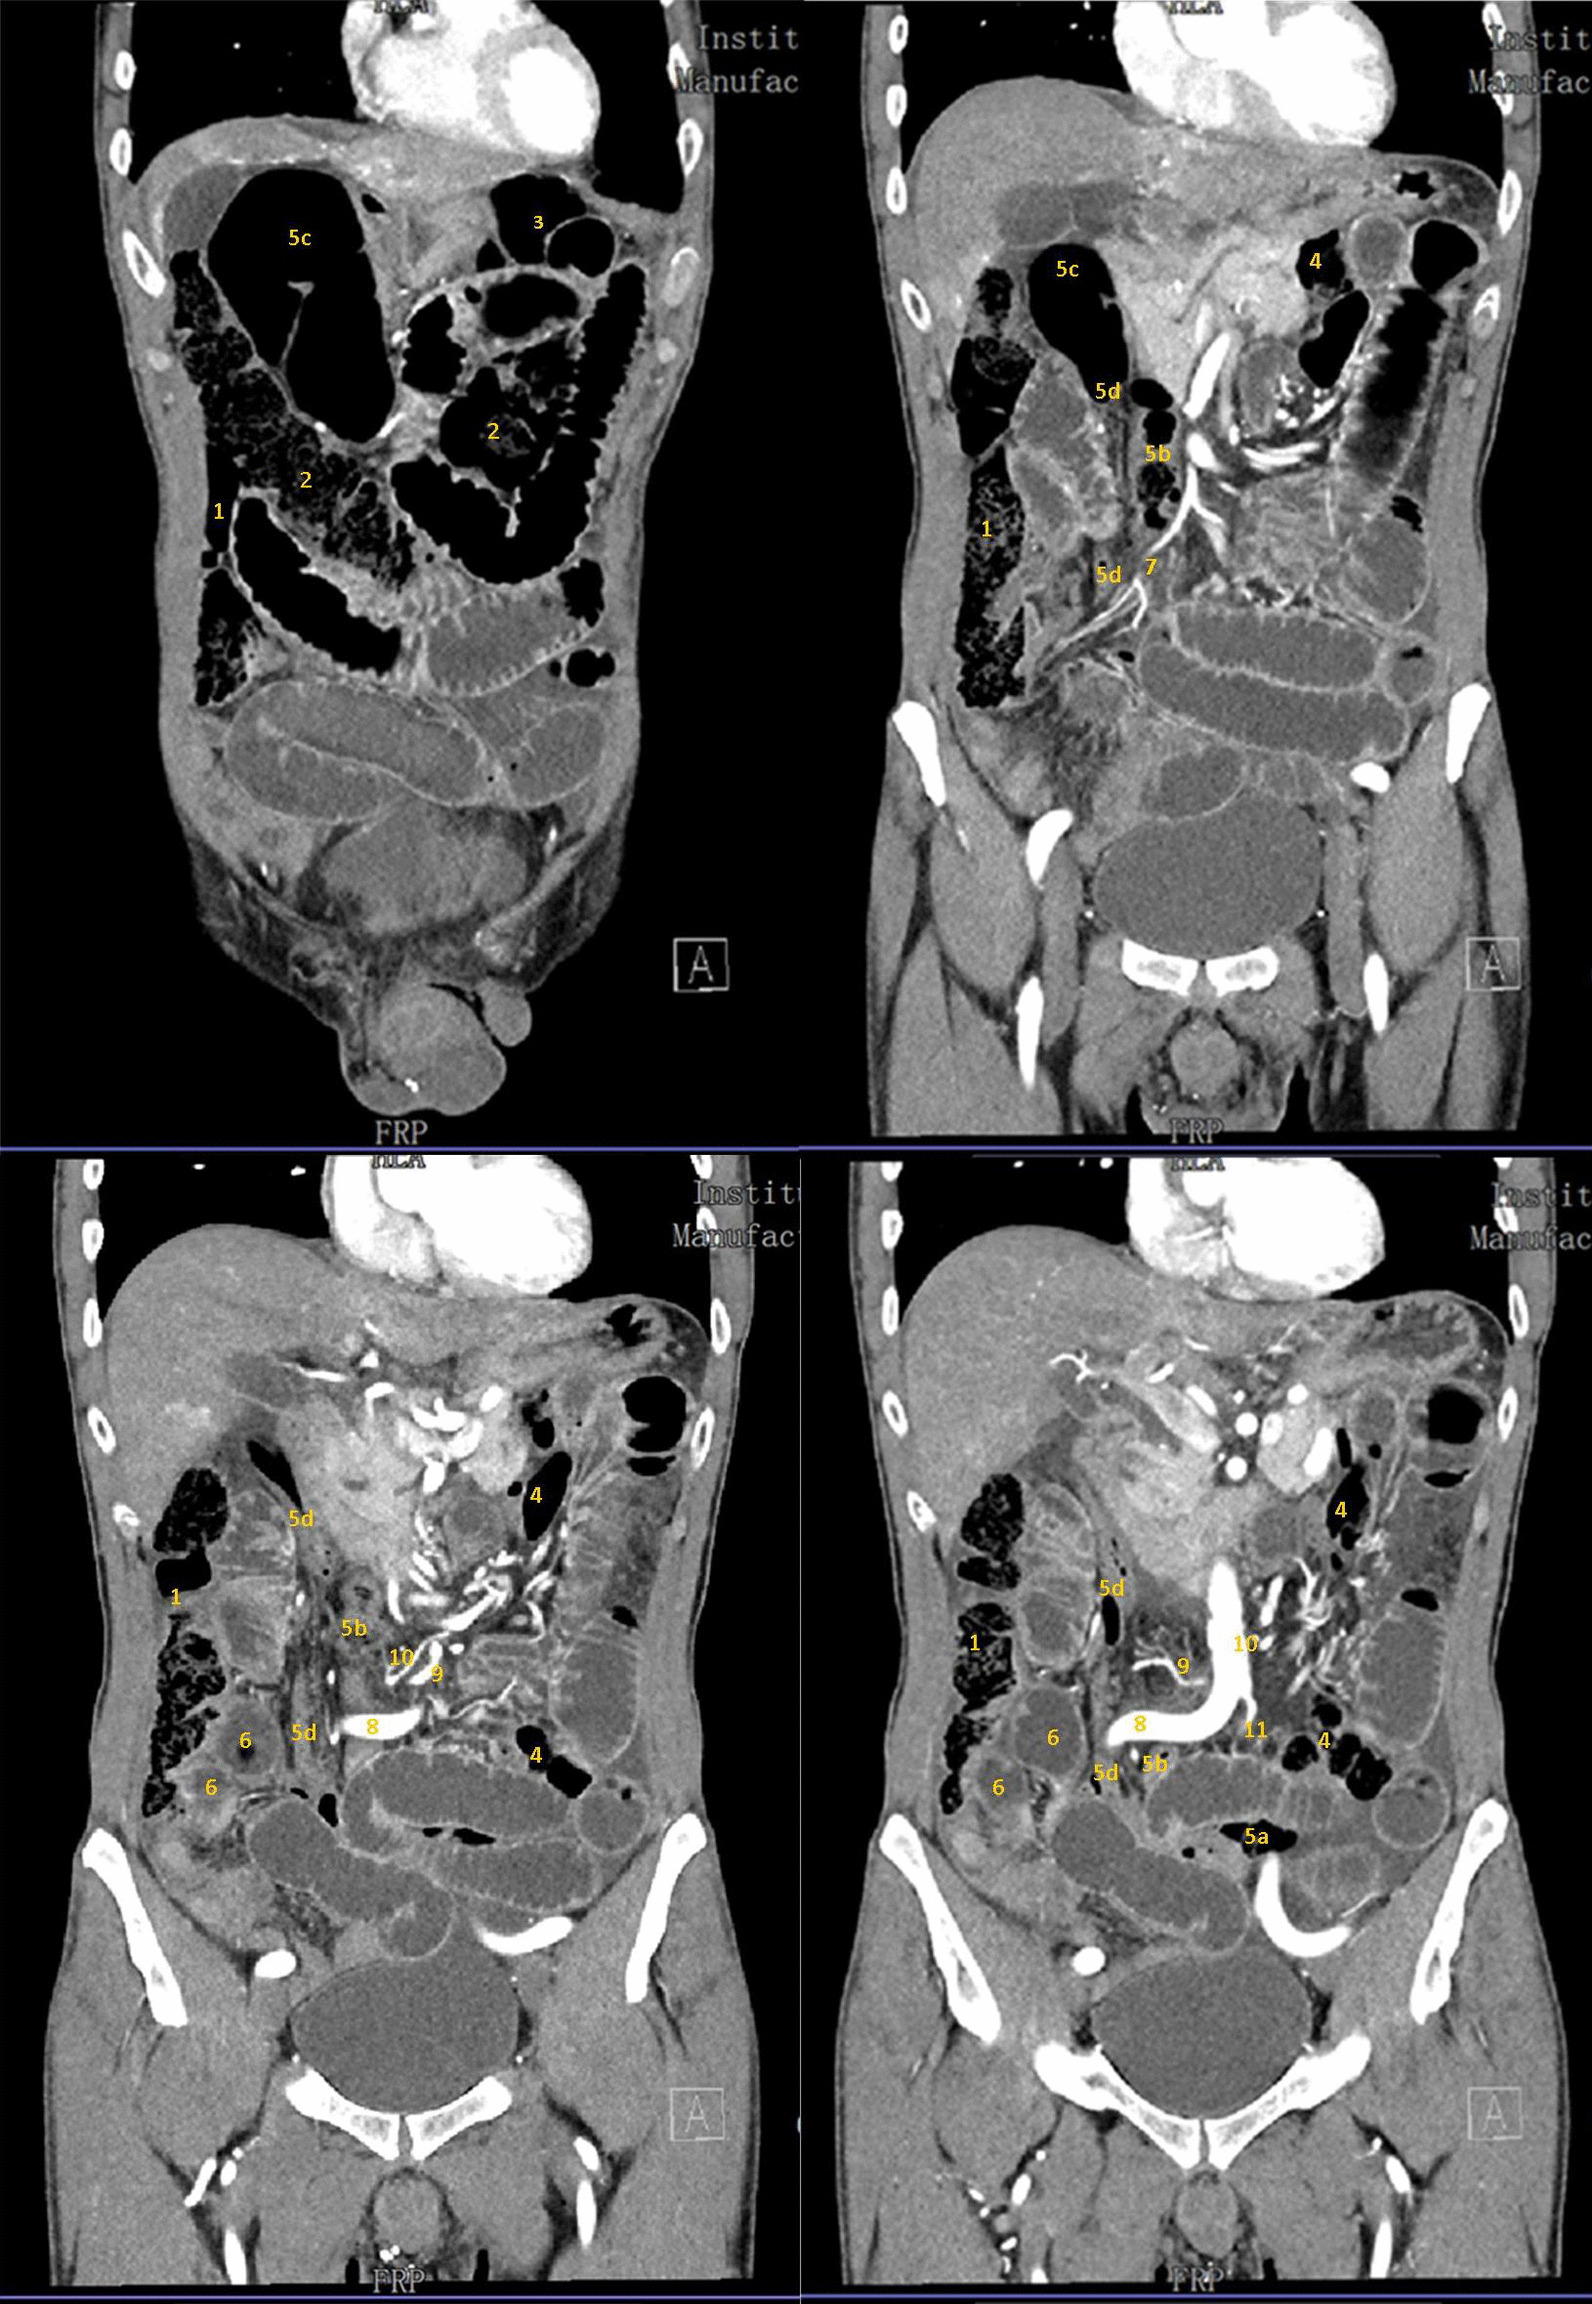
Coronal view of abdominal Computed tomography scan (from anterior to posterior): (1) ascending colon; (2) transverse colon; (3) splenic flexure; (4) descending colon; (5) sigmoid colon: a first, b second, c third, d fourth part; (6) incarcerated ileal loop; (7) ileocolic artery; (8) right common iliac artery; (9) inferior mesenteric artery; (10) left colic artery; (11) left renal artery
